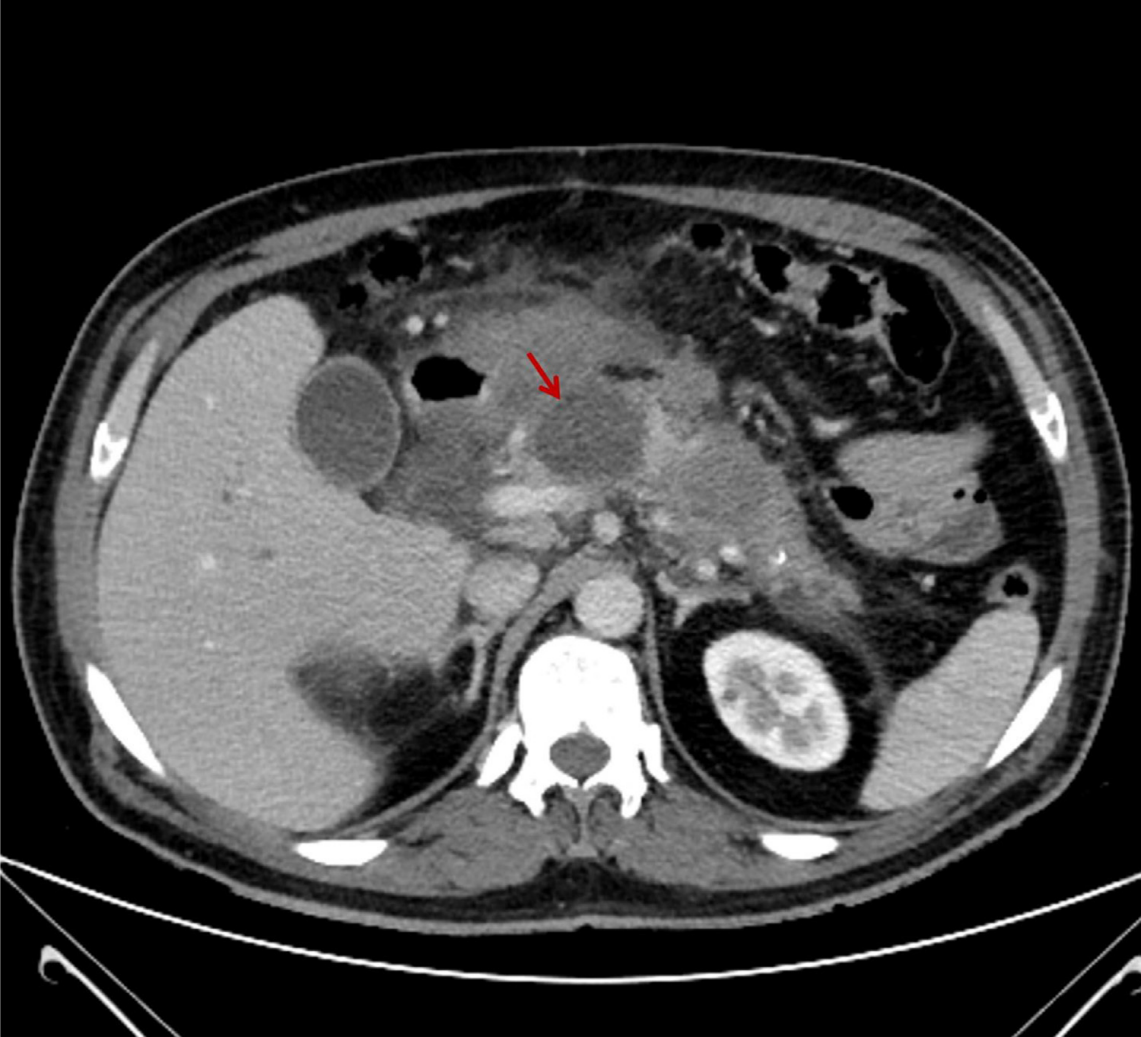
Abdominal CT scan–Axial view: The pancreas shows diffuse inhomogeneous parenchymal density and enhancement. Some areas of decreased enhancement are seen in the pancreatic tail, associated with peri-pancreatic fat stranding, suggestive of acute necrotizing pancreatitis. Evidence of pseudocyst formation is noted in the pancreas, measuring 38 × 43 mm (red arrow).
